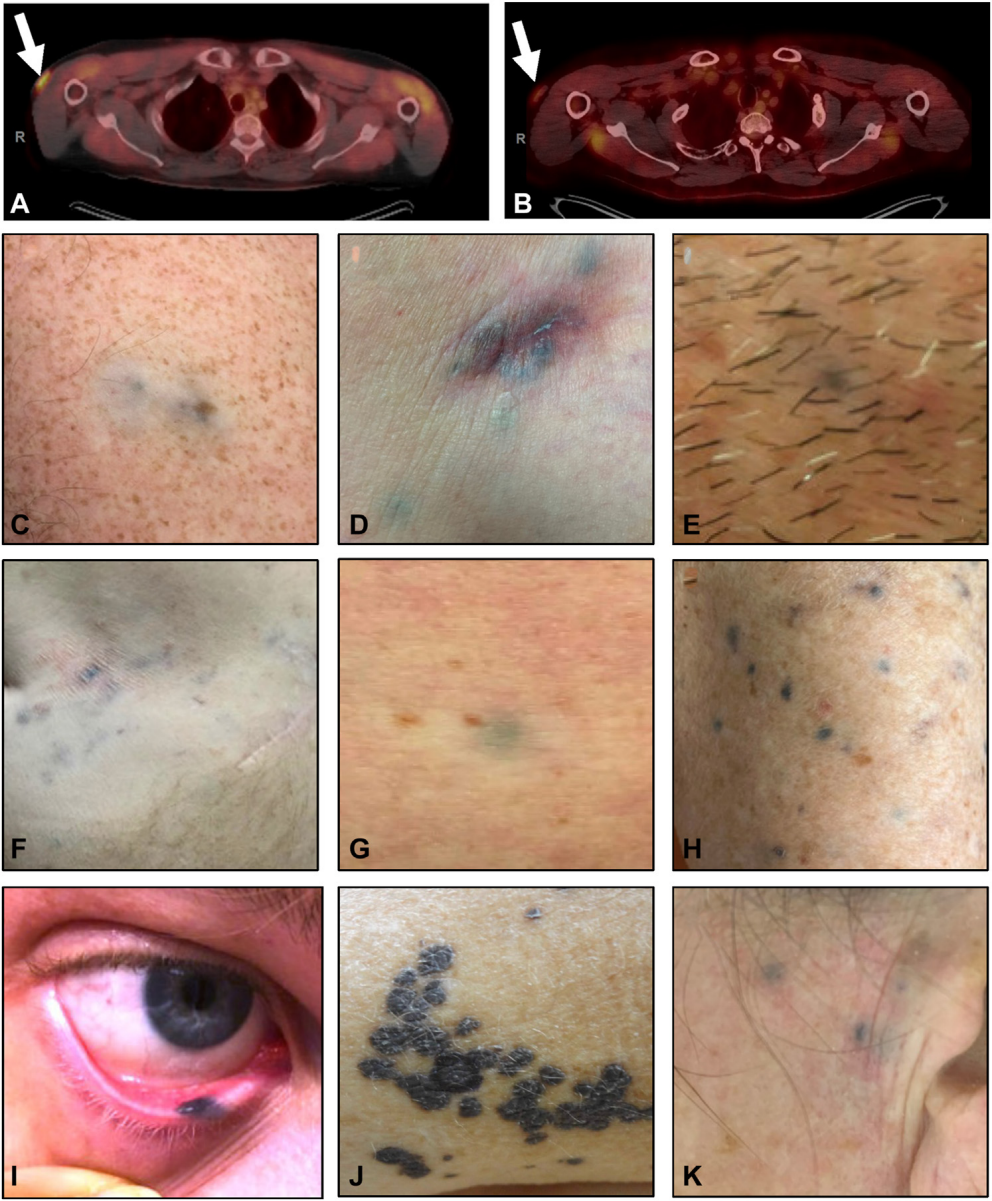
Varying clinical presentations of tumoral melanosis after immunotherapy in patients with metastatic melanoma and correlative findings on positron emission tomography-computed tomography (PET-CT). A-C, Sequential PET-CT images of a metastatic melanoma nodule (*white arrow*) that went from high fluorodeoxyglucose (FDG)-avidity (A) to normal FDG-avidity (B) 1.5 years after the start of immunotherapy, resulting in biopsy-proven tumoral melanosis pictured in (C). D-K, Varying clinical presentations of tumoral melanosis in patients with metastatic melanoma after systemic immunotherapy at our institution.
